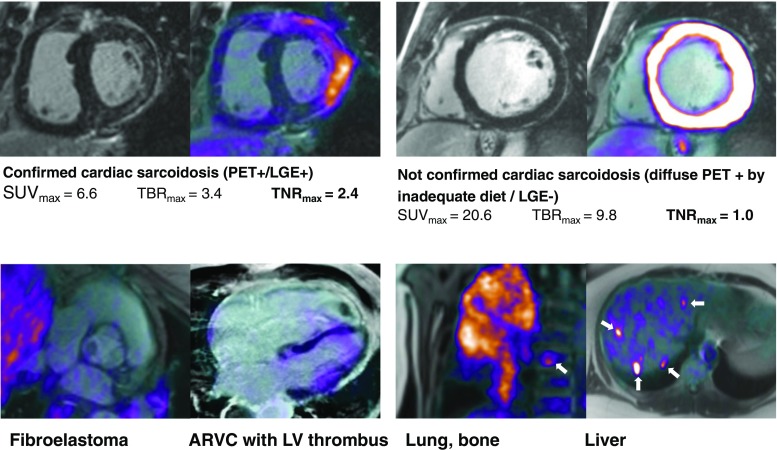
^18^F-FDG PET/MRI images in patients with suspected cardiac sarcoidosis. *ARVC* Arrhythmogenic right ventricular cardiomyopathy, *LGE* Late gadolinium enhancement, *LV* Left ventricle
